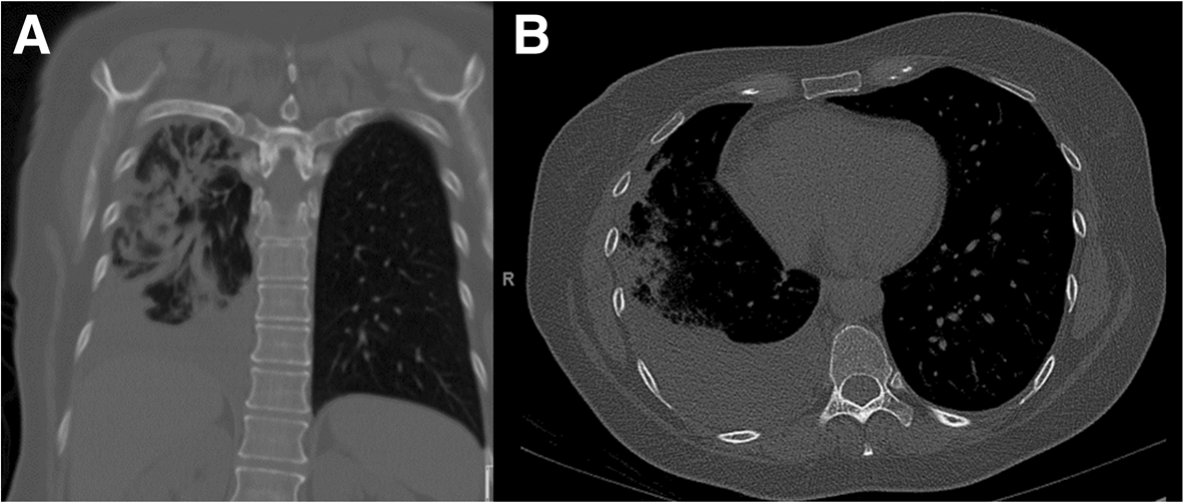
Chest computed tomography showing moderate-sized pleural effusion in the right chest ("a" and "b")
